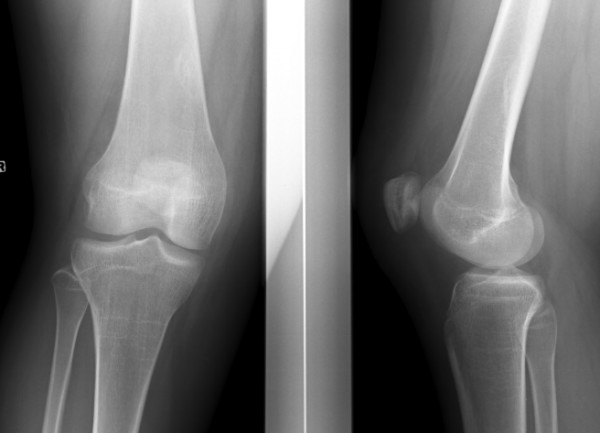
Postoperative radiograph: the normal patellar-femoral relationship has been restored.
